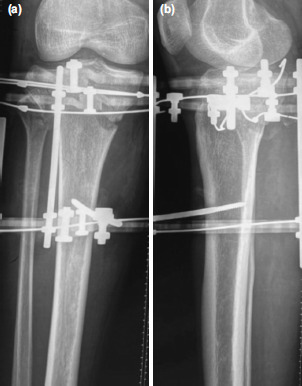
Case No.7, post-operative radiographs. (a) Anteroposterior view showing olive wore fixation through fibular head for buttressing of latera condyle. (b) Lateral view.
